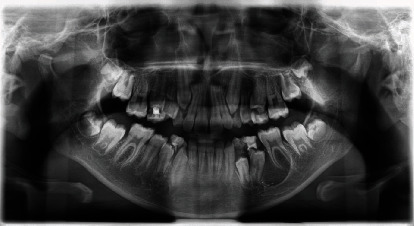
Pre-Op Orthopantomograph showing a well-defined radiolucent lesion in the left posterior mandible.
